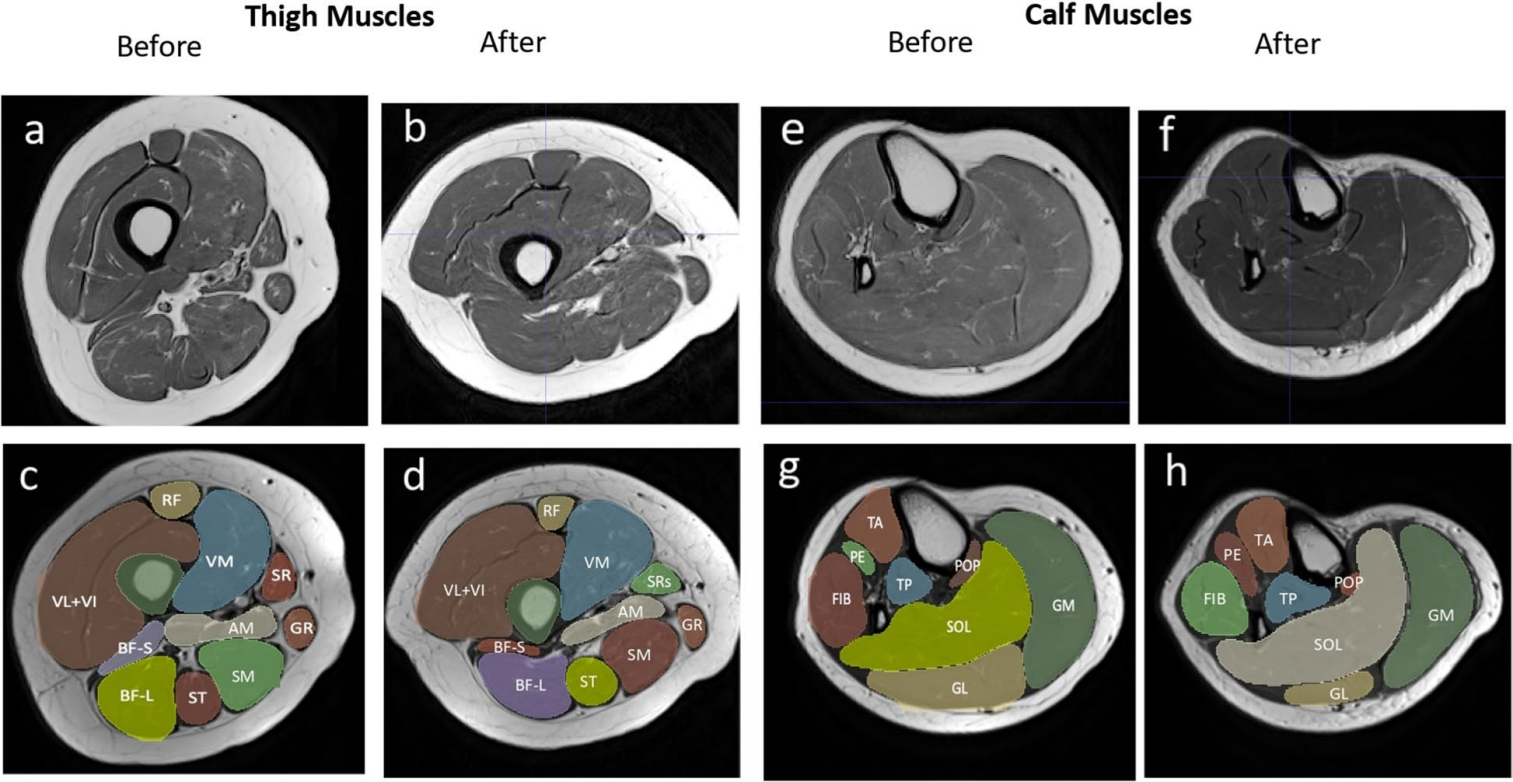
Proton Density MRI of Thigh and Calf Muscles Pre- and Post-Injury with Immobilization: Proton density MRI of thigh (a-d) and calf (e–h) region before (a, c, e, g) and after (b, d, f, h) injury and immobilization. Images were segmented to identify the following muscles: rectus femoris (RF), vastus lateralis + intermedius (VL + VI), vastus medialis (VM), biceps femoris short head (BF-S), biceps femoris long head (BF-L), semimembranosus (SM), semitendinosus (ST), sartorius (SR), gracilis (GR), adductor magnus (AM), tibialis anterior (TA), phalangeal extensors: extensors digitorum and hallucis (PE), peroneals a.k.a. fibulari (FIB), tibialis posterior (TP), popliteus (POP), soleus (SOL), medial gastrocnemius (MG), and lateral gastrocnemius (LG). Note: The pre- and post-injury segmentation images shown are not from the same slice in the limb. Reported quantitative volume analysis provides accurate assessments of changes
